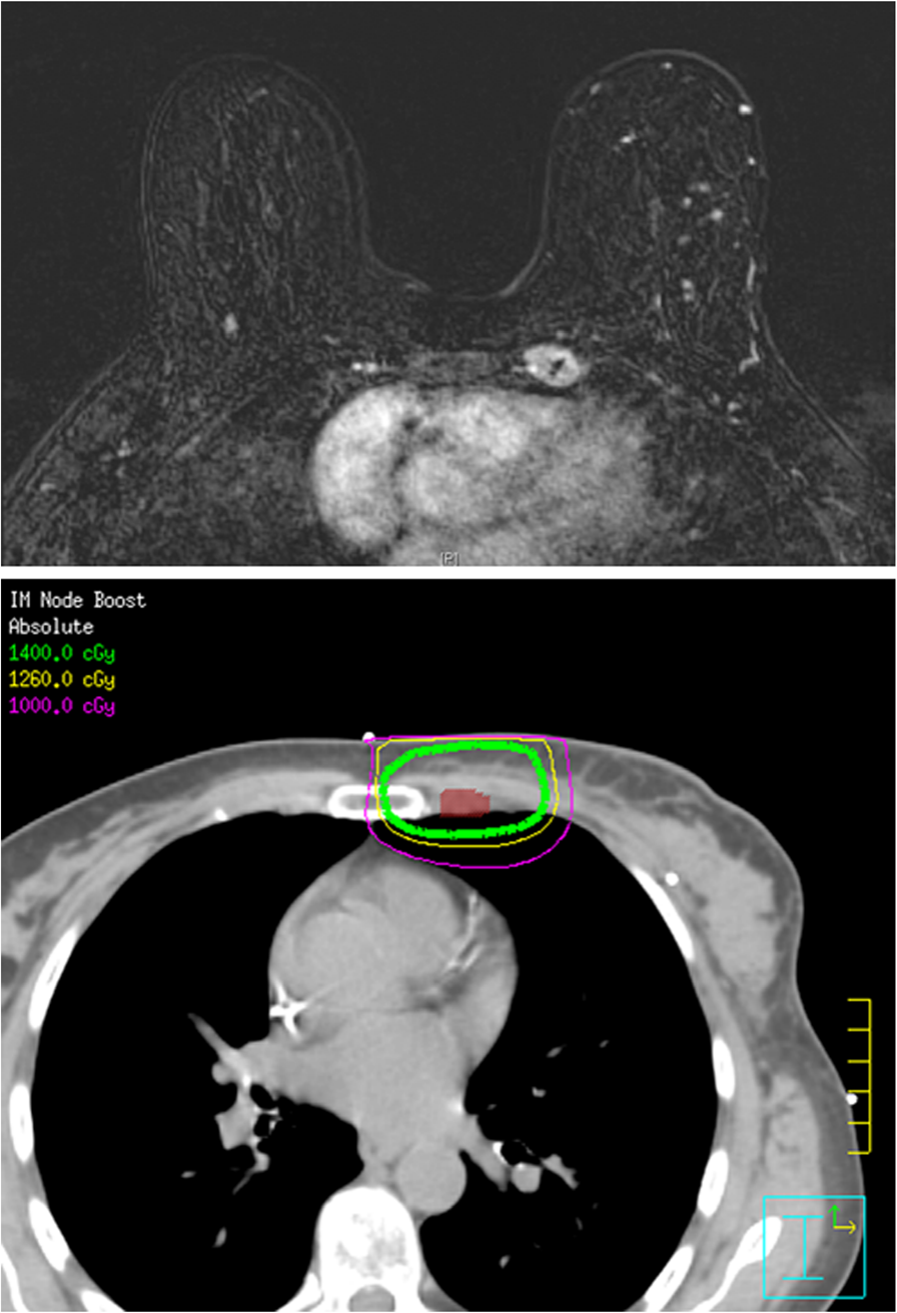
Representative MRI and treatment plan. Representative internal mammary node identified on MRI (Top) and treatment plan of dedicated 14Gy boost (Bottom). Maroon contour = IM node GTV
